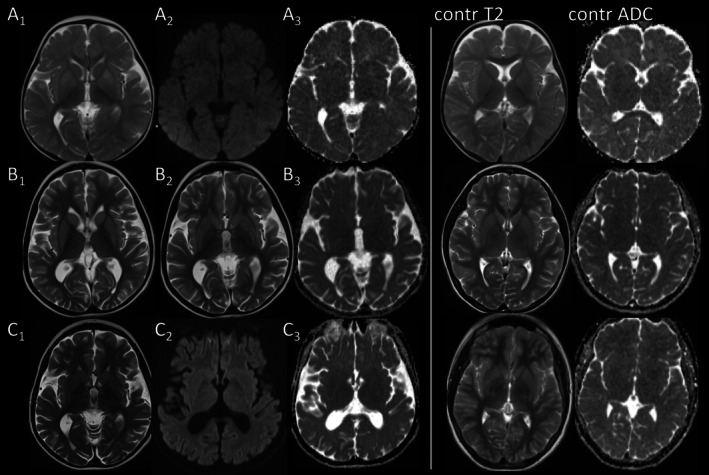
Striatal and reversible pallidal involvement in p4. (A) Swelling and T2‐hyperintensity of putamen and pallidum in p4 at 1.1 years on day 8 of acute metabolic decompensation, but without restricted diffusion. (B) At 12,1 years (FU3) there is new caudate involvement, again without restricted diffusion on day 1 of acute metabolic decompensation with impaired consciousness. Pallidal T2‐hyperintensity had already resolved at prior MRI at 1.9 years (not shown). (C) At 13.2 years (FU5) signal of basal ganglia has normalized with signal of striatum slightly less than that of insular cortex (compare with age‐matched control). Diffusion remains normal, generalized atrophy has increased. (T2w: A_1_–C_1_, B_2_, DWI: A_2_, C_2_, ADC‐maps: A_3_–C_3_.)
